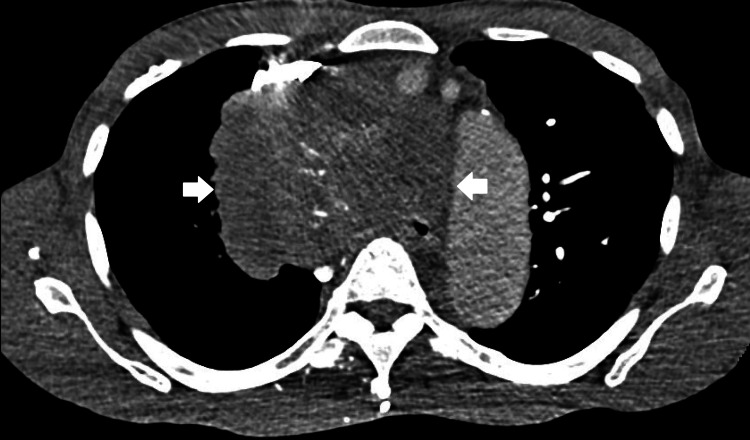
Mediastinal CT scan images. White arrows mark the large mediastinal mass with partial internal calcifications.
